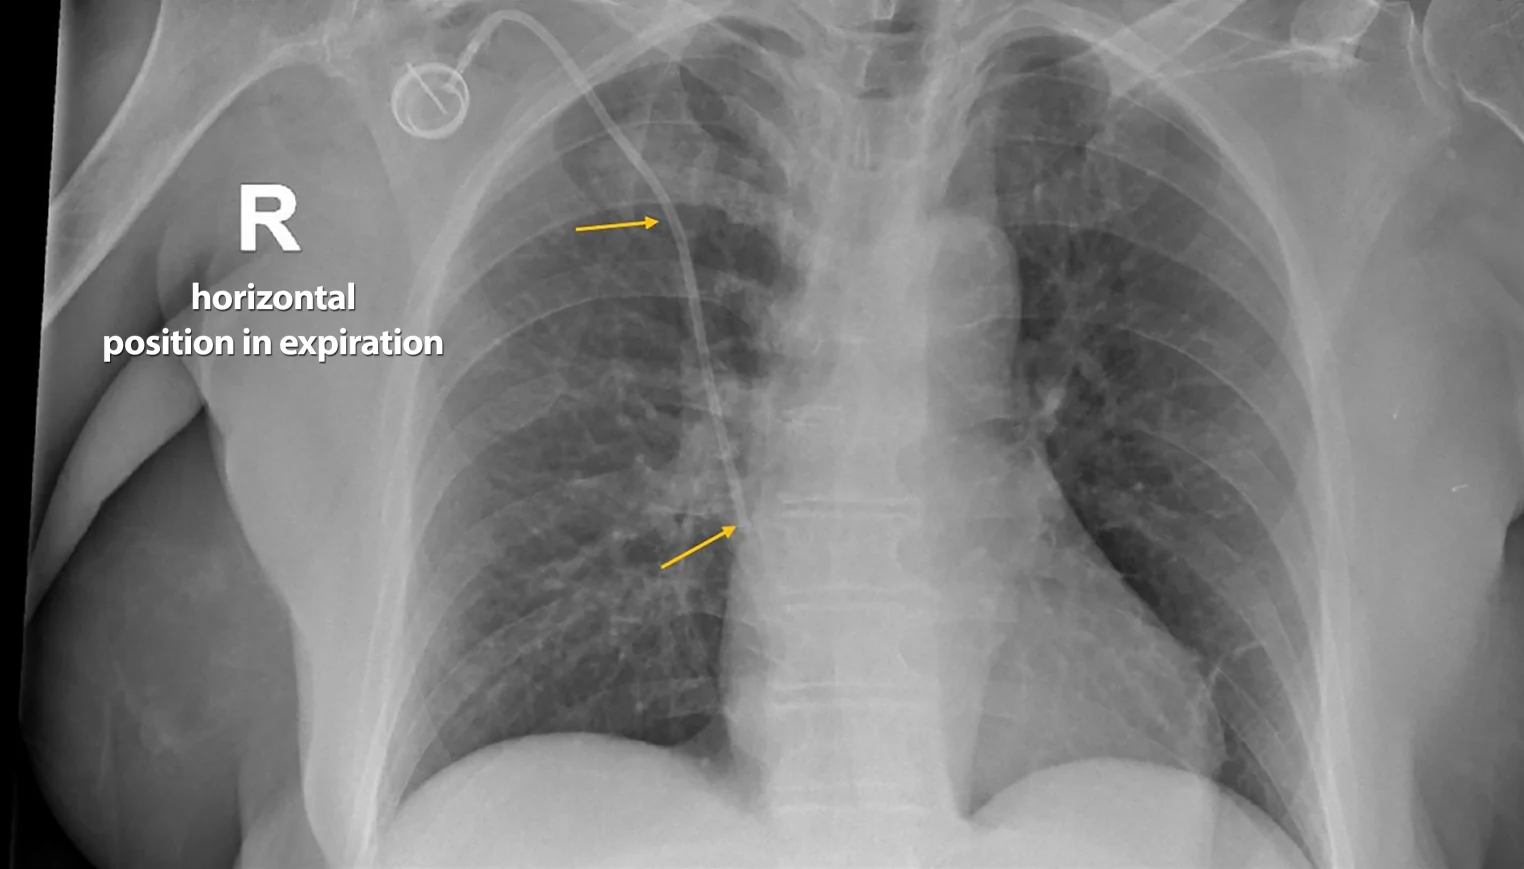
The postoperative chest X-ray, which already showed the intrapulmonary malposition of the Port-a-Cath catheter
